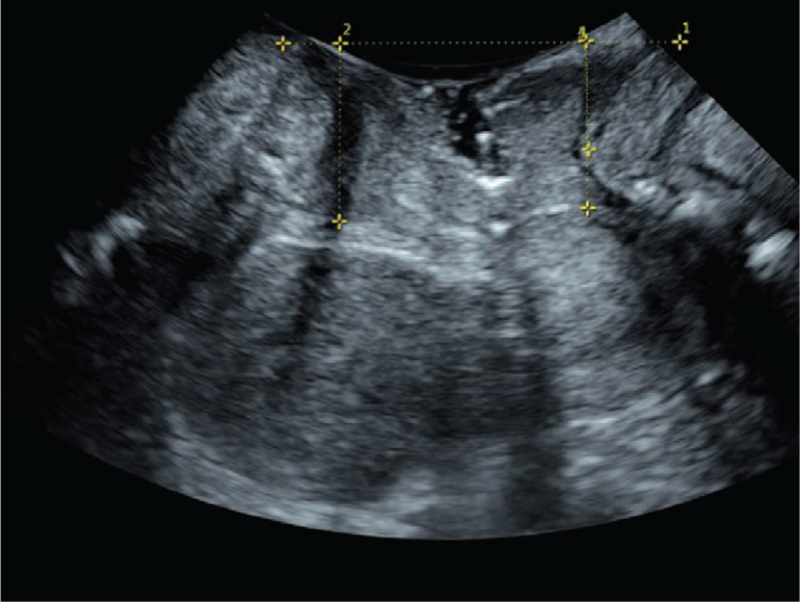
Ultrasonic image taken in resting state. ^∗^ Represents that there is a significant difference in the decrease of group B compared with group A (*P* < .05). Number 1 represents draw a horizontal line through the lower edge of the pubic symphysis in a resting state. Number 2 to 4 represent the distance measurements from the lowest point of the bladder neck (number 2), the lowest edge of the cervix (number 3), and the lowest point of the rectal ampulla (number 4).
